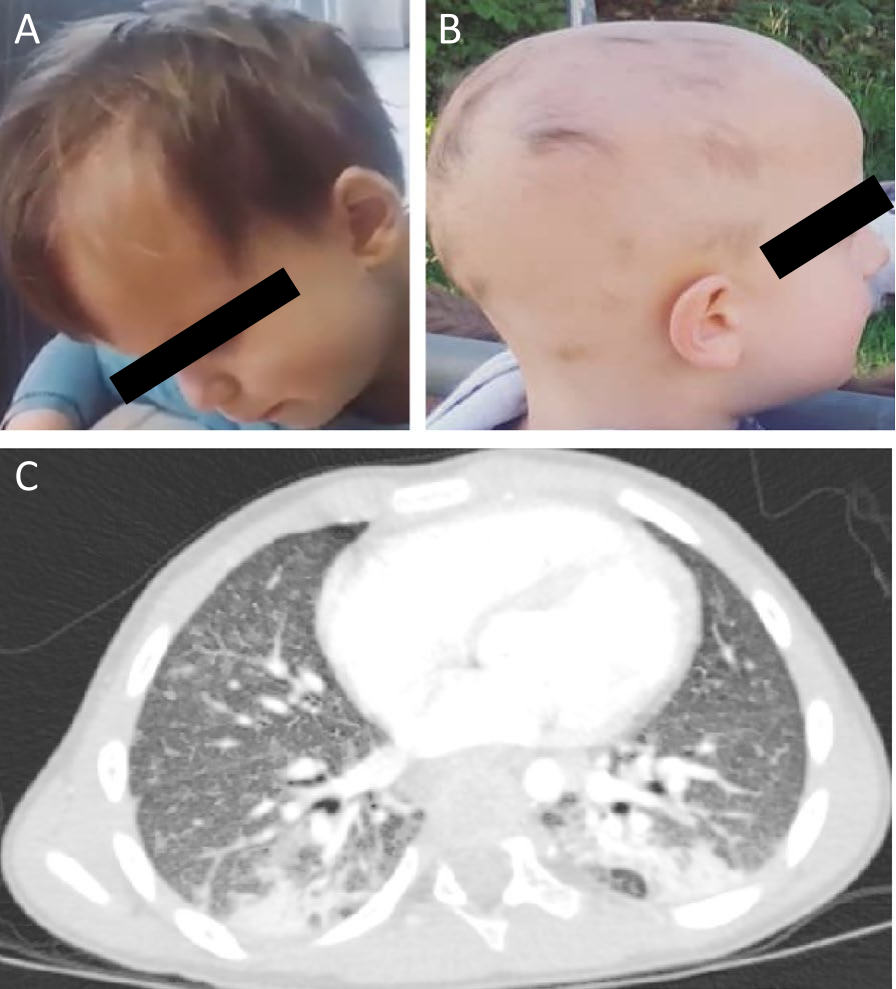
Clinical findings in index patient P1. A. Alopecia areata starting from the left forehead. B. Alopecia totalis with only isolated hair tufts. C. Computed tomography of the chest showing interstitial vasculitic lung disease with ground glass opacities and caudal small nodular parenchymal lesions. Basal bronchopneumonic infiltrates are present on both sides
